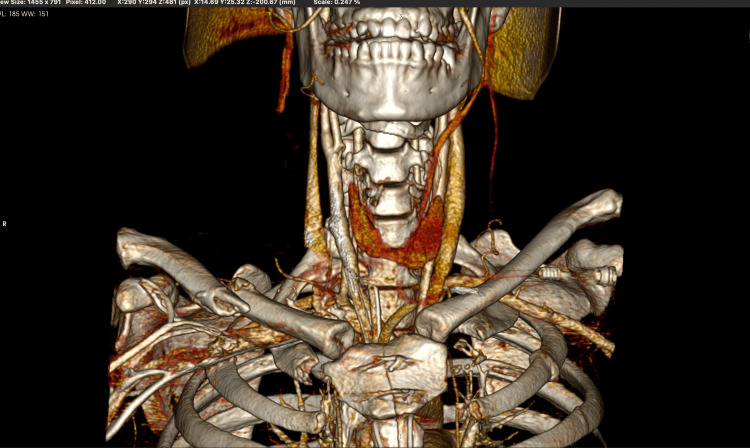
A postoperative 3D reconstruction based on a CT presenting complete revascularization of the right CCA CCA: common carotid artery
